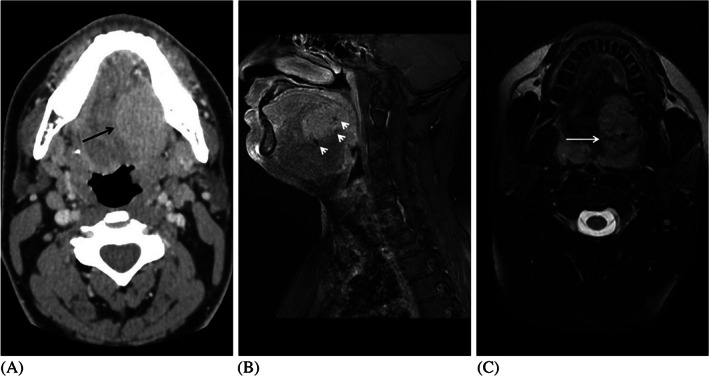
imaging findings of ASPS. A, Axial contrast enhanced computed tomography demonstrates a homogenous well defined hypervascular lesion in left posterior tongue (white arrow). B, Sagittal enhanced T1 weighted image demonstrates a tongue mass with homogenous pattern of contrast en‐hancement with flow void structures (white arrows). C, Axial unenhanced T2 weighted image demonstrates a hyperintense tongue mass with flow voids (white long arrow) and areas of slightly higher intensities (black short arrows) due to tumor necrosis
